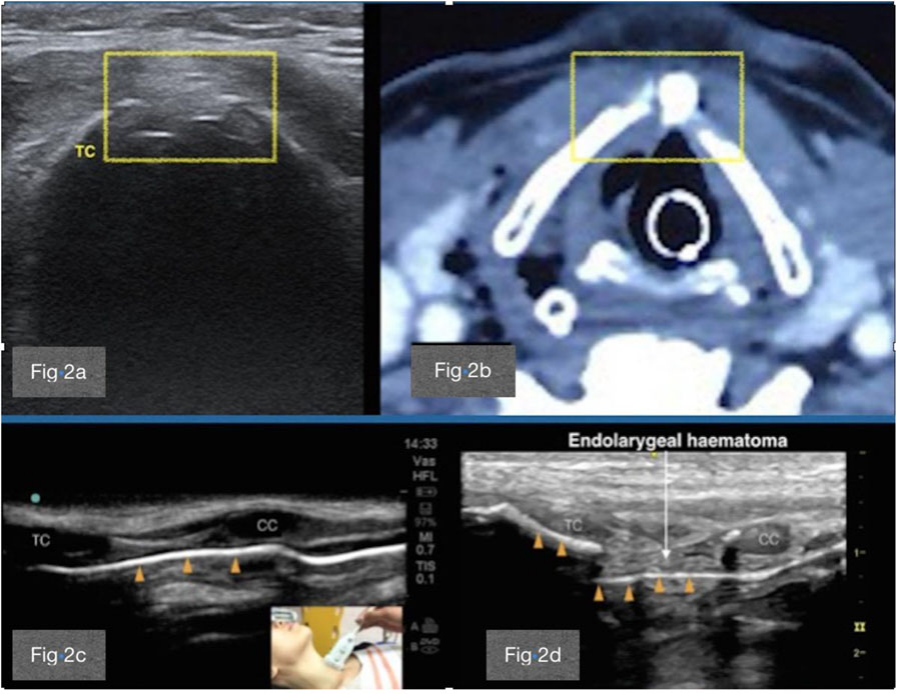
Figure 2a: Figure of POCUS airway of displaced thyroid cartilage fracture and disruption of air mucosal. Figure 2b: A Computerised Tomography (CT) scan image shown defect in posterolateral wall of trachea with fracture of right anterior lamina of thyroid cartilage and superior cornu of left thyroid cartilage in relation to the image in POCUS. TC- thyroid cartilage. Figure 2c – Normal sonoanatomy in longitudinal scan showing continuous intact air mucosal as shown by yellow arrowhead. Figure 2d- POCUS airway in longitudinal scan showing disruption of air mucosal interface and displaced thyroid cartilage as shown by yellow arrowhead and formation of endolaryngeal haematoma. TC – thyroid cartilage; CC- cricoid cartilage
